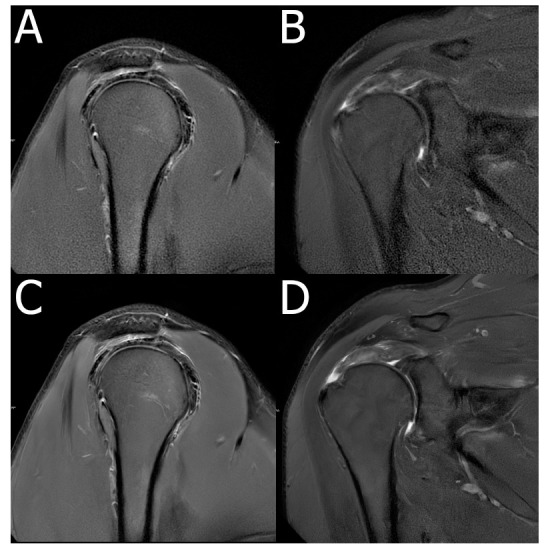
MRI with and without the Deep Resolve algorithm. Sagittal (A) and coronal (B) PROPELLER accelerated FS PDWI images. The addition of Deep Resolve algorithms (C, D) increases SNR, reduces motion artifacts, and yields overall better image quality.
